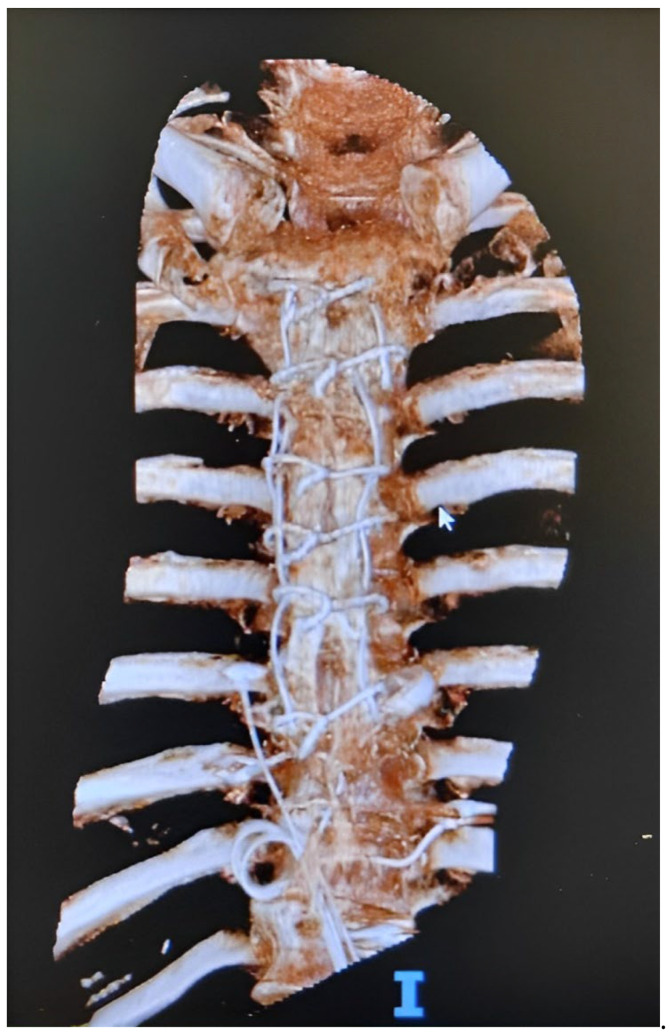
Preoperative 3D CT scan—outcomes of sternotomy and sternal wiring from the previous surgery are visible.
